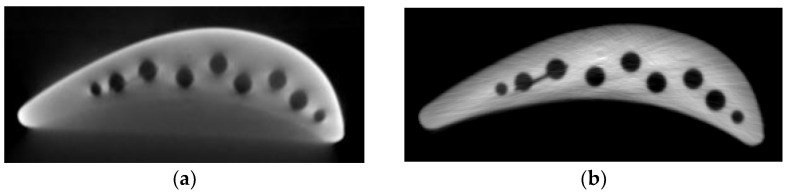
Comparison of reconstructed CT images of turbine blades before and after correction. (a) Image with beam hardening artifacts; (b) corrected image.
